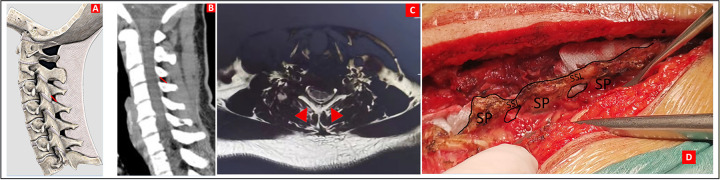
(A–B) The absence of ISL; (C) Cervical MRI image: fatty space under the cervical paravertebral muscles (red triangles); (D) The absence of the ISL was also observed in the cervicothoracic surgery (C6–T2). SP, spinous process; SSL, supraspinous ligament.
